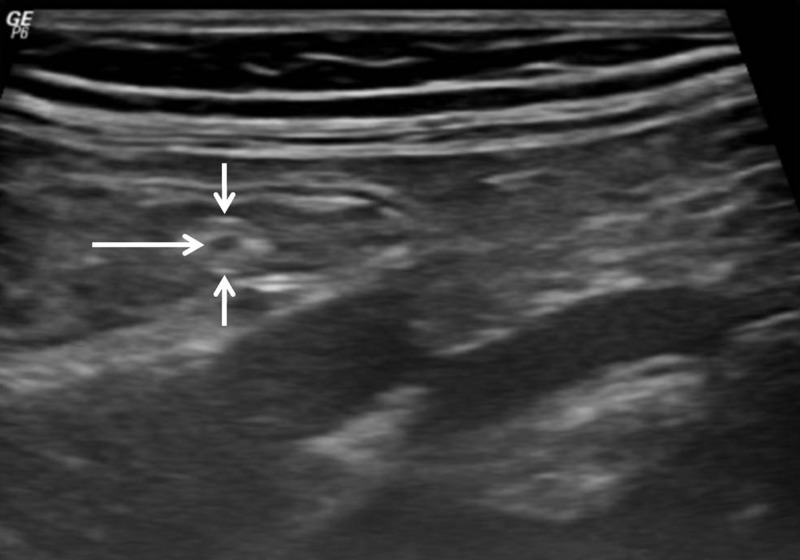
(A, B) Sonographic images show linear parallel echogenic structures that look like “railway tract” on longitudinal imaging and “bull's eye” on transverse imaging (small white arrows). There is no posterior acoustic shadowing. These tubular structures showed active movement on real time sonography, thereby confirming worm infestation. The central anechoic linear area represents the digestive tract of the worm (long white arrow).
